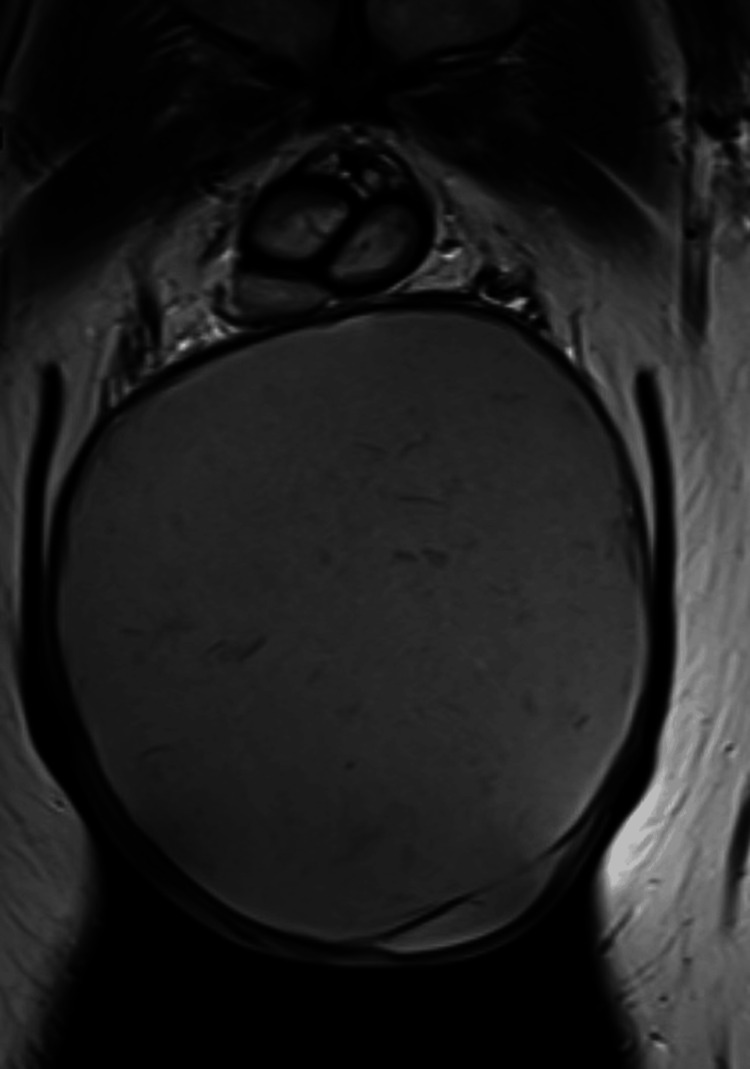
Coronal T2-weighted magnetic resonance imaging of the pelvis showing a giant, well-defined, thin-walled, homogeneous hyperintense cystic lesion occupying almost the entire scrotal sac, causing marked compression and displacement of the adjacent scrotal structures without obvious solid components or septations.
